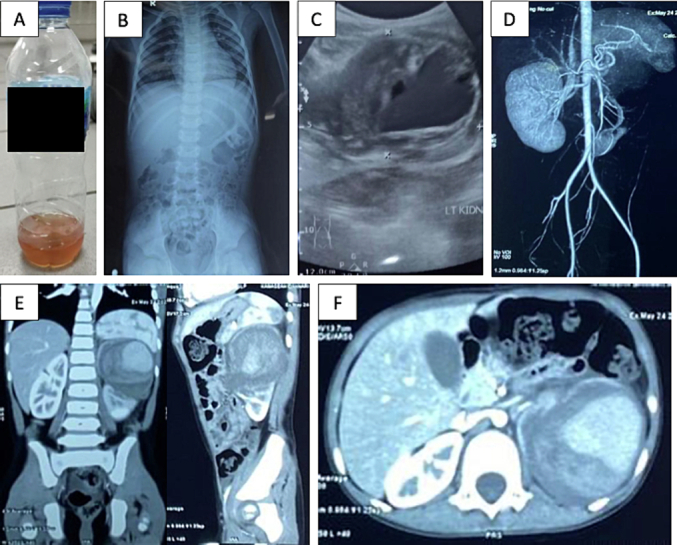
(A) Physical examination showed no hematuria; (B), Babygram results were expected; (C) ultrasound imaging revealed an aneurysm in the left kidney; (D, E, F) Abdominal CT angiography confirmed the presence of a hematoma at the upper pole with a pseudoaneurysm connected to a branch of the left renal artery.
